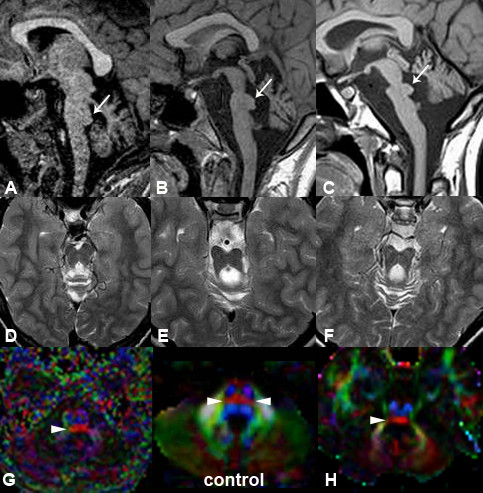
Neuroimaging of PTCD patients. MRI and DTI at 1.5 T of patient 1 (A, D, G), patient 2 (B, E) and patient 3 (C, F, H). In all the patients, sagittal T1-weighted images (A, B, C) show the typical "cap" (arrows) in the pontine tegmentum protruding into the fourth ventricle, diagnostic for the malformation. Note also the flat ventral pons and dysplastic cerebellar vermis. T2-weighted images (D, E, F) at the level of the isthmus reveal a "molar tooth-like" appearance, with laterally displaced superior cerebellar peduncles. Colour-coded fractional anisotropy maps of patient 1 (G) and patient 3 (H) are shown with standard DTI conventions (blue for superior↔inferior direction perpendicular to plane of section, red for right↔left direction, green for anterior↔posterior direction). The tegmental "cap" (arrowhead) is a bundle of transversely oriented fibres, possibly representing "ectopic" pontine transverse fibres, normally located in the midpons (double arrowheads in "control").
